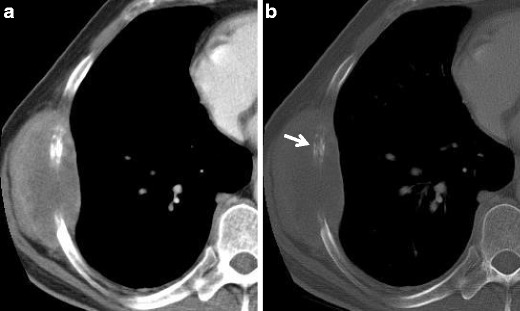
Sarcomatoid mesothelioma; axial view contrast-enhanced CT scan: soft tissue (a) and bones (b) window images showing a large thoracic wall mass with inhomogeneous enhancement, involving the lateral arch of a rib, that appears eroded (*white arrow*), a typical infiltration sign
